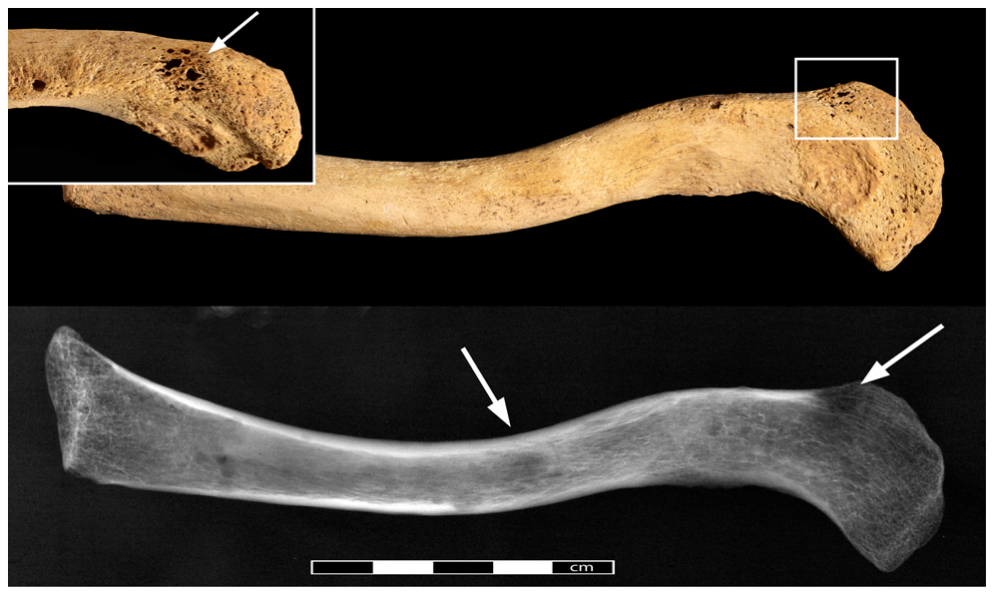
The left clavicle of Sk244-8. General view of the left clavicle with the pathological lesions indicated by arrows. Radiograph of the same bone on the bottom. Insert shows close-up of the lesion on the superior margin indicated by rectangle.
